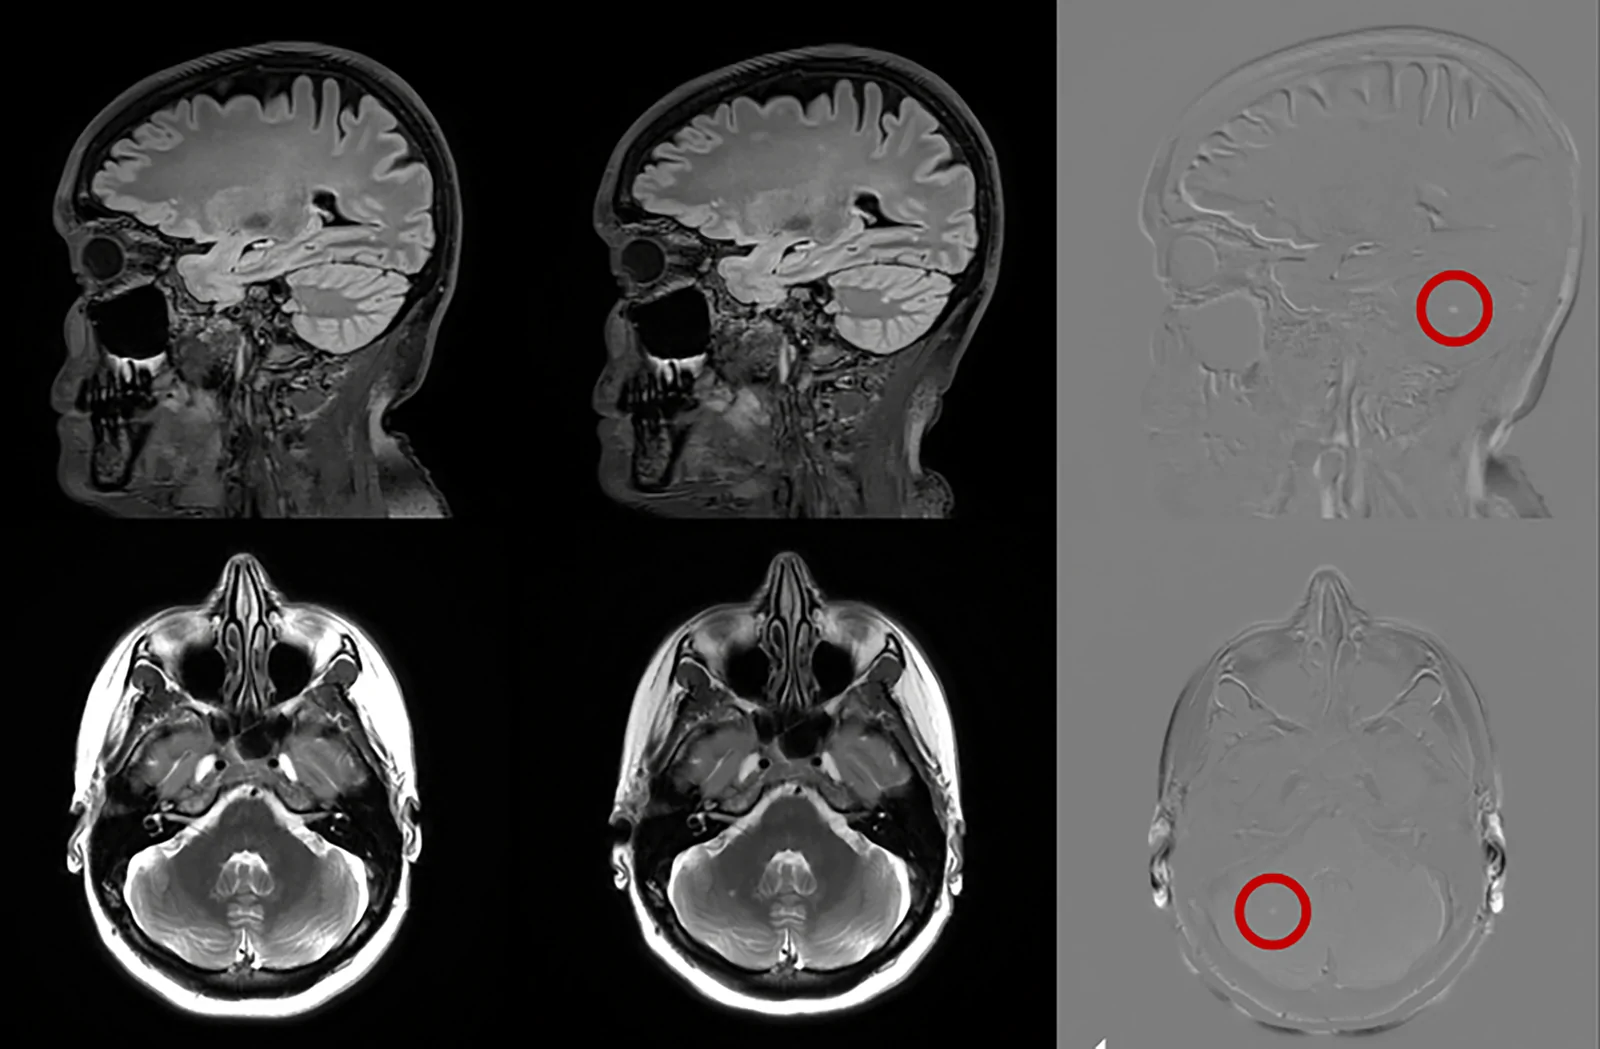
MS disease monitoring by MRI reveals a clinically silent, new infratentorial T2-hyperintense lesion on 3D T2w FLAIR and axial T2w TSE at follow-up (middle panel) compared to baseline (left panel). Consistent acquisition (same scanner and coil, same sequence parameters) of 3D sequences facilitates reliable detection of both new and enlarging T2-hyperintense lesions using longitudinal subtraction (right panel) [86]
